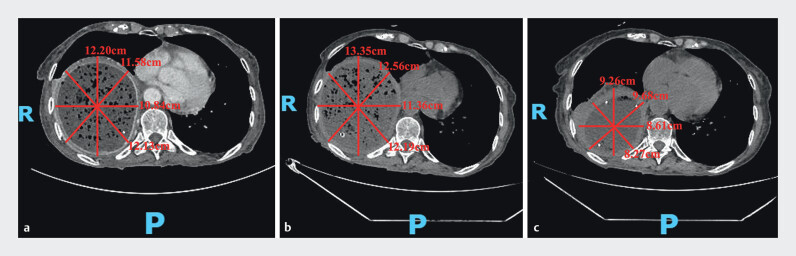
Computed tomography images obtained at the same cross-sectional level.
